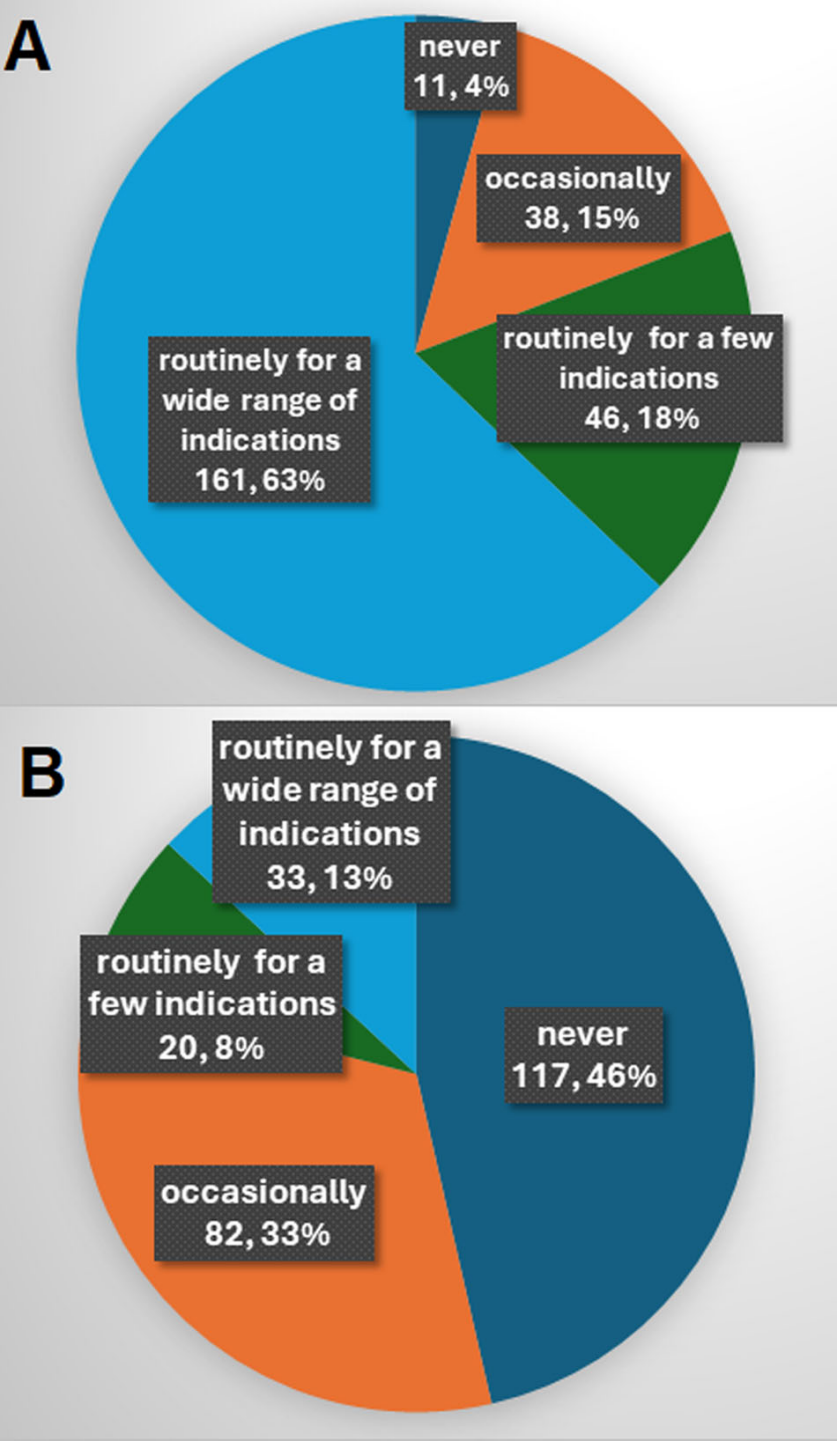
Pie charts indicating the number and proportion of all institutions (*n* = 256) where (A) DWI and (B) DCE-PWI are never acquired, occasionally acquired, routinely acquired for a narrow range of indications or routinely acquired for a wide range of indications
